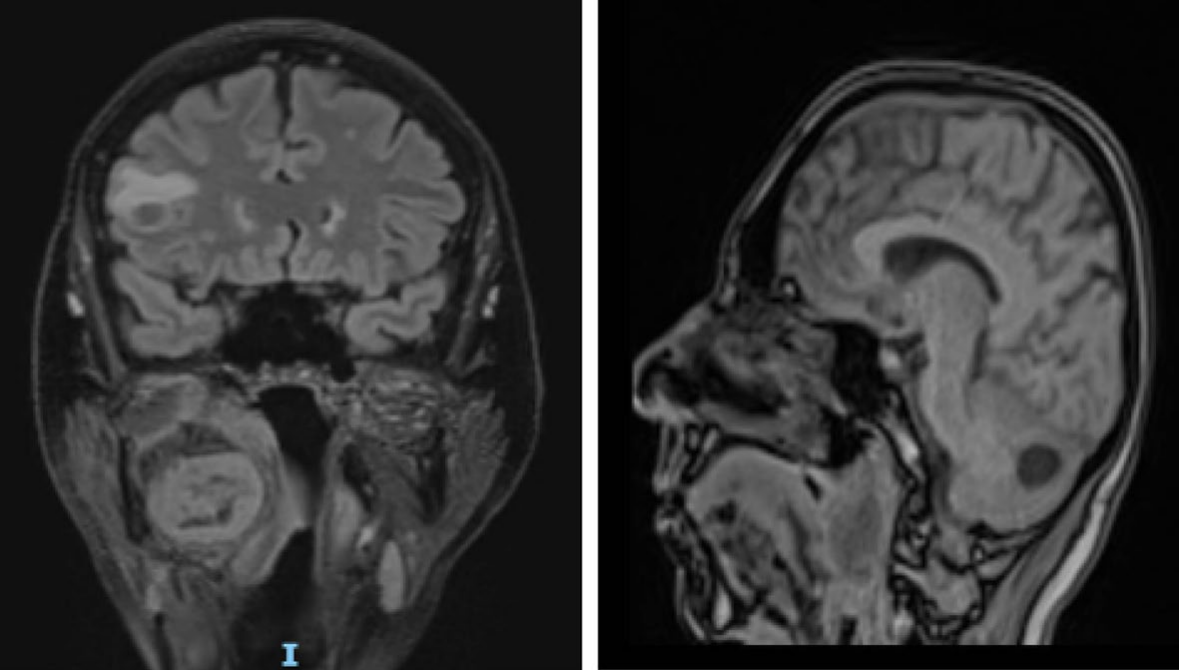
Brain MRI with sagittal section (T2_space 3D dark fluid sequences). Detail on the parapharyngeal formation with evident mass effect on the trachea; detail on the cerebellar formation
